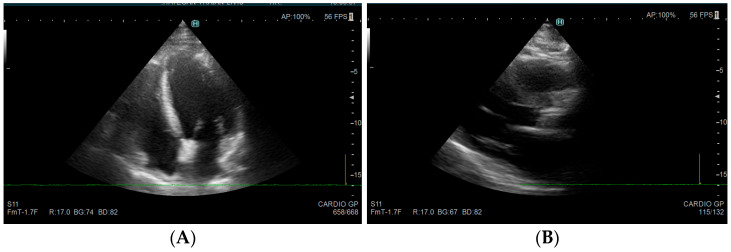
Transthoracic echocardiographic appearance of advanced mitral and aortic valve calcification in chronic kidney disease. (A) Parasternal long-axis view showing marked echogenic thickening of the mitral valve leaflets, consistent with severe calcific degeneration. The mobility of the valve is reduced, and acoustic shadowing is present. (B) Apical view revealing calcified aortic cusps with restricted opening during systole, indicative of hemodynamically significant aortic stenosis. Source: Images obtained at Spitalul de Urgență Deva, Romania. Used with institutional permission.
